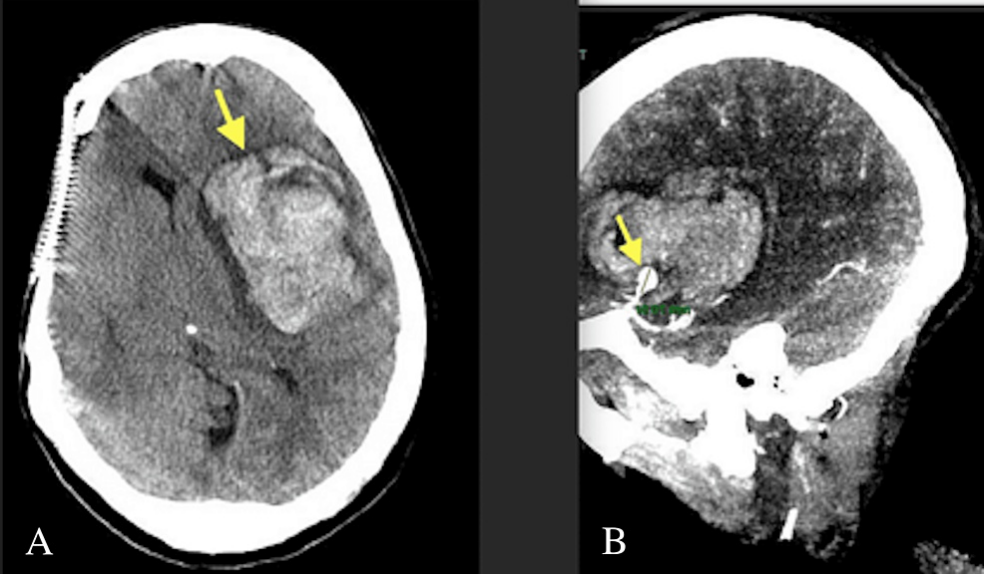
CT head performed after neurological deterioration (A) Arrow indicating intracerebral hemorrhage due to a ruptured mycotic aneurysm. (B) Arrow indicating increased 10 mm mycotic aneurysm (from baseline 3 mm, not shown) after rupture.
